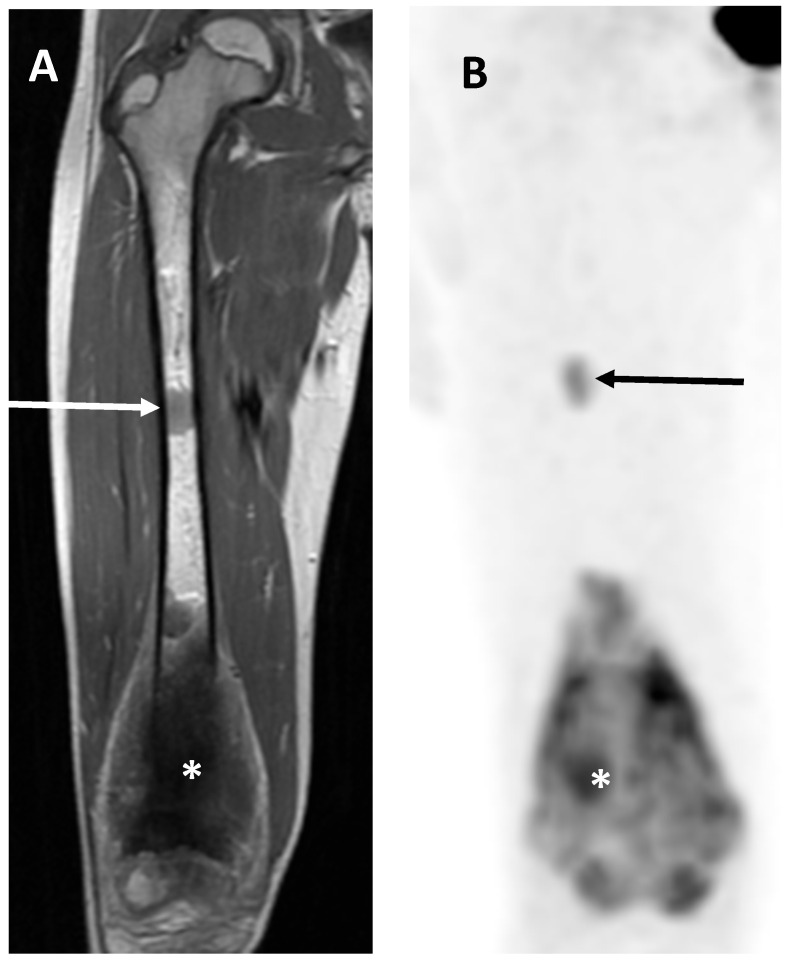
Skip metastasis. Coronal T1-weighted imaging (WI) (A) and view of 18F-FDG PET/CT (B) of 9-year-old female showing principal osteosarcoma mass of right distal femur (asterisks) together with proximal ‘skip metastasis’ (arrows).
